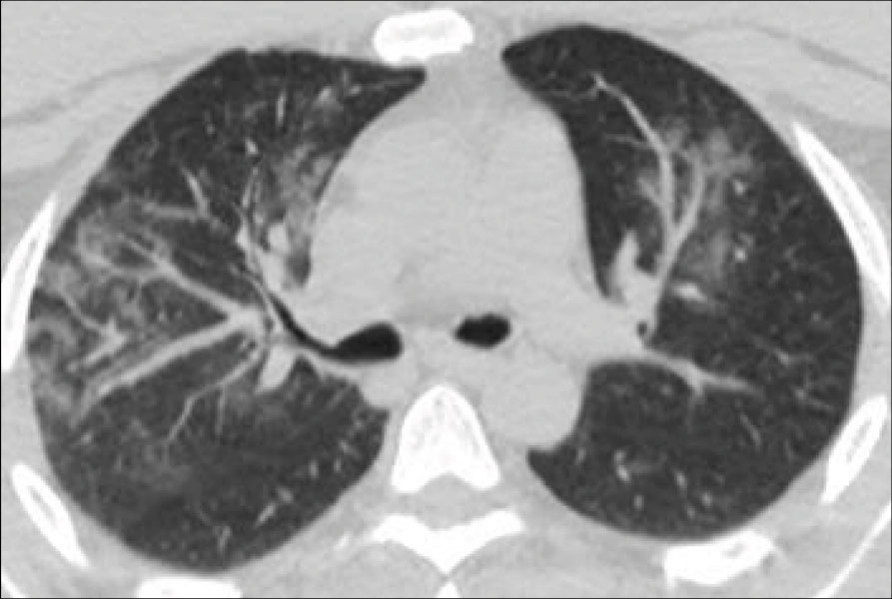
A 40-year-old male presenting with headache, low-grade fever, and cough. His PaO_2_/FiO_2_ ratio was 350 mmHg. Axial thin-section unenhanced CT image shows bilateral upper lobe vascular enlargement within surrounding dense ground-glass opacities with interstitial thickening
